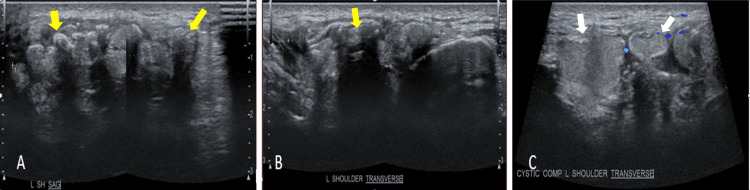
Ultrasound of the left shoulder (A, B) Well-defined complex masses predominantly composed of multiple echogenicity exhibiting posterior acoustic shadowing (yellow arrows) and areas of (C) cystic foci with midlevel echoes exhibiting Brownian motion upon compression (white arrows). The mass has a conglomerate measurement of about 5.1x4.3x1.5 cm (CCWAP).
